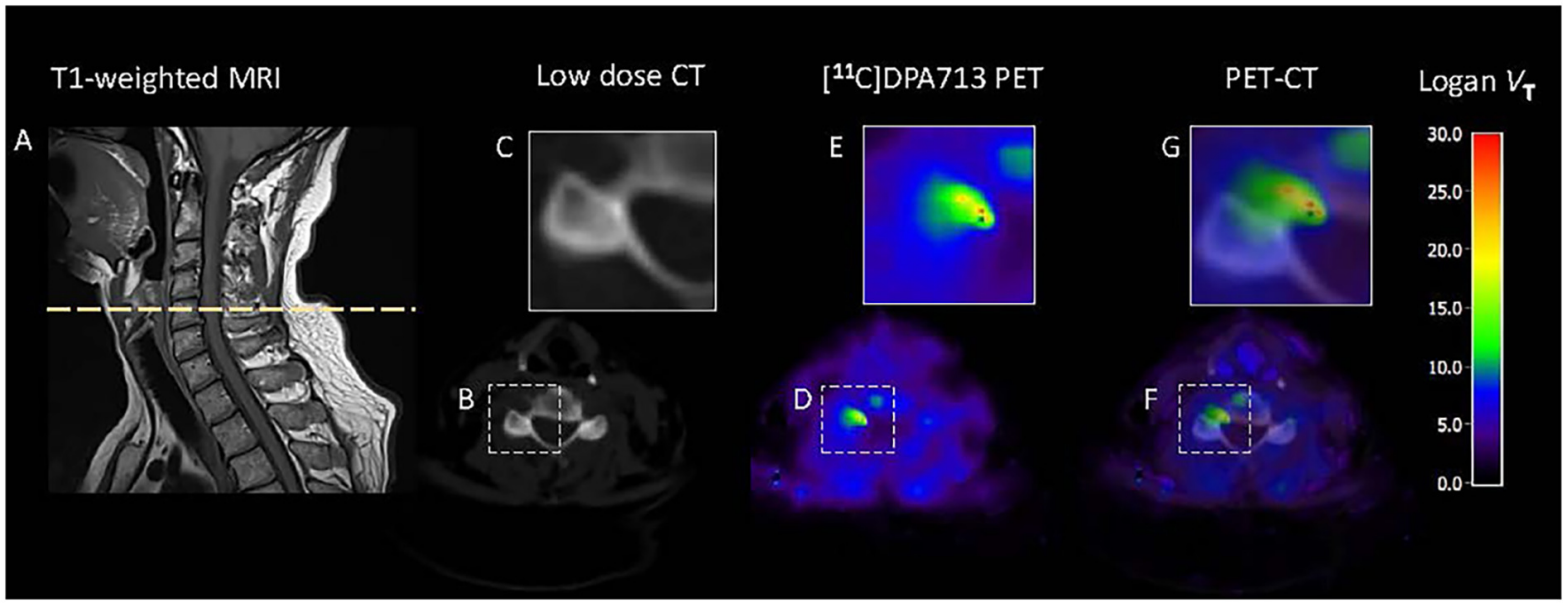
Graphical summary. Neuroinflammation in a patient with painful cervical radiculopathy C6. Patient was a mixed-affinity binder for TSPO polymorphism. To quantify [^11^C]DPA713 binding at the neuroforamina and spinal cord, a single tissue compartment (1T2k) model with image derived input function was used. For visualization purposes, volume of distribution (*V*_T_) images were generated using Logan plot analysis, with a time threshold of t* = 30 min. (A) T1-weighted MRI. The dotted line indicates the cross-sectional area used for the CT and PET analysis. (B) Low-dose CT cross-sectional image, with (C) a zoomed view at the neuroforamina. (D) A parametric cross-sectional image of [^11^C]DPA713 binding, with (E) a zoomed view at the neuroforamina. Higher volume of distribution (*V*_T_) indicates more tracer binding, suggesting higher levels of neuroinflammation. (F) CT and PET images merged with (G) a zoomed view at the affected neuroforamina.
